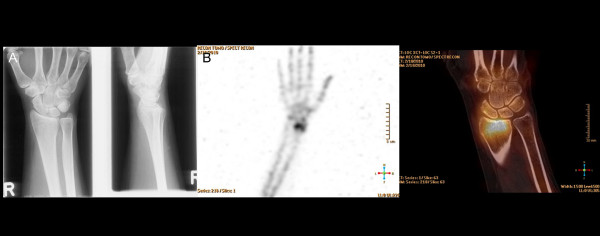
A 32-year-old female with persistent dorsoradial wrist pain after distortion. (A) No bone lesion was detected on plain radiographs. (B) High radioisotope uptake in the distal radial epiphysis with bumpy RC joint surface, representing persistent bone remodeling after consolidated distal radial fracture (left: 3D-SPECT, right: fusion SPECT/CT). Occupational therapy was initiated after SPECT/CT.
